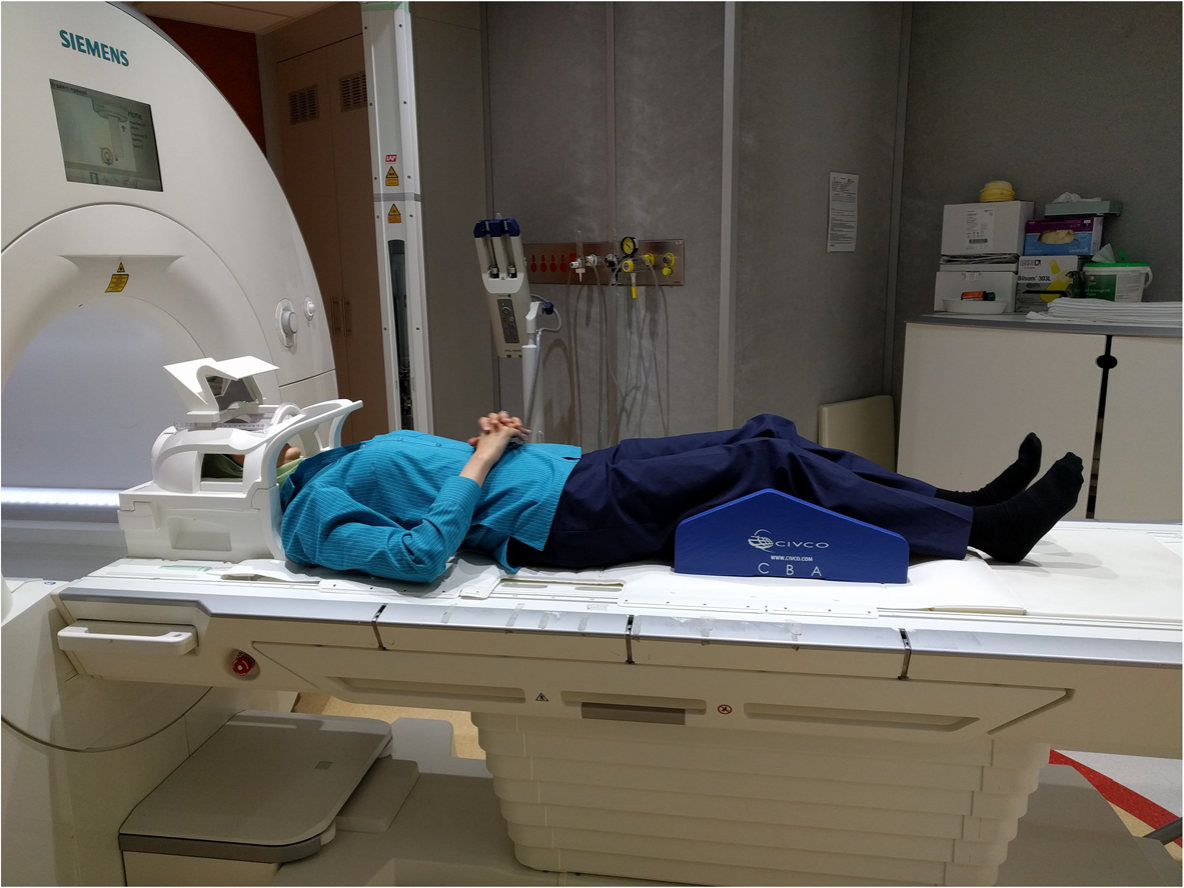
Research scan patient setup for MRI in MPHNC study
